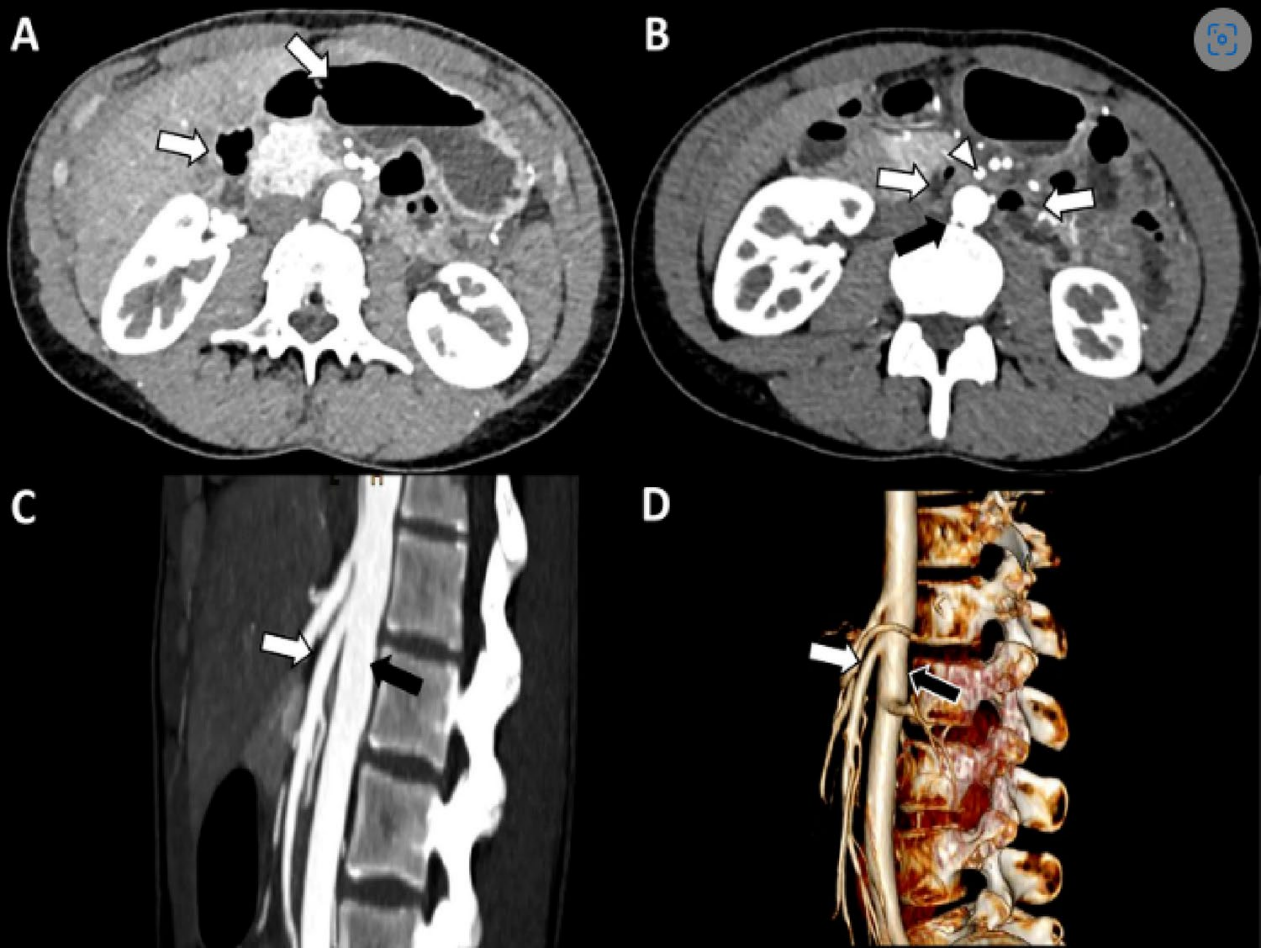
Superior mesenteric artery syndrome. Axial contrast-enhanced CT images at the level of the upper abdomen. A Mild distension of the stomach and proximal duodenum (white arrows). B Compression of the third portion of the duodenum (white arrows) between the superior mesenteric artery (white arrowhead) and abdominal aorta (black arrow). C Sagittal images show reduced angle and distance between the superior mesenteric artery (white arrow) and abdominal aorta (black arrow) (aortomesenteric angle of 18°, aortomesenteric distance of 5 mm). D Sagittal 3D reconstruction again shows the reduced angle between the superior mesenteric artery (white arrow) and abdominal aorta (black arrow)
